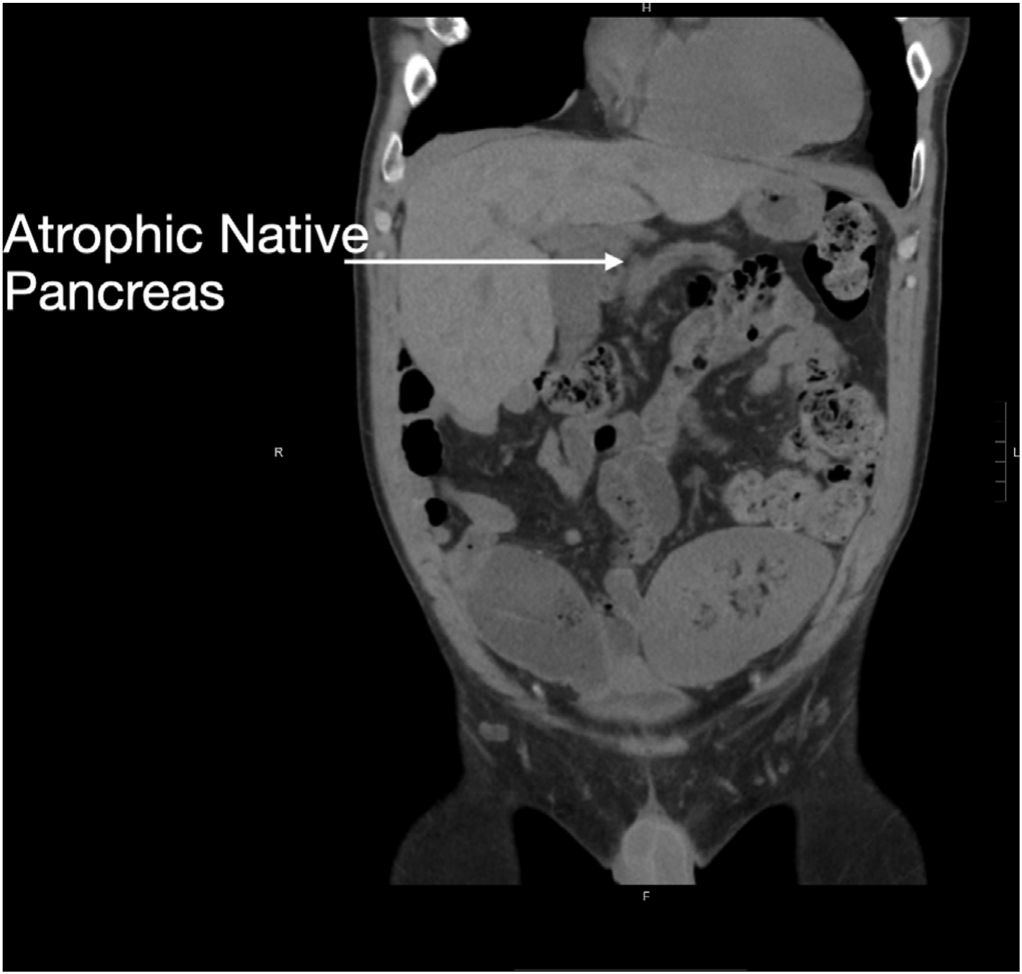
Intravenous contrast-enhanced computed tomography abdomen-pelvis demonstrating atrophic but otherwise normal-appearing native pancreas.
